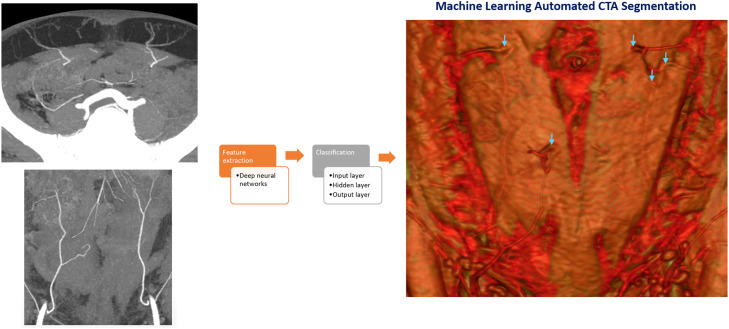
Use of machine learning properties of computational models and algorithms to automatically conduct computed tomography angiogram segmentation.
